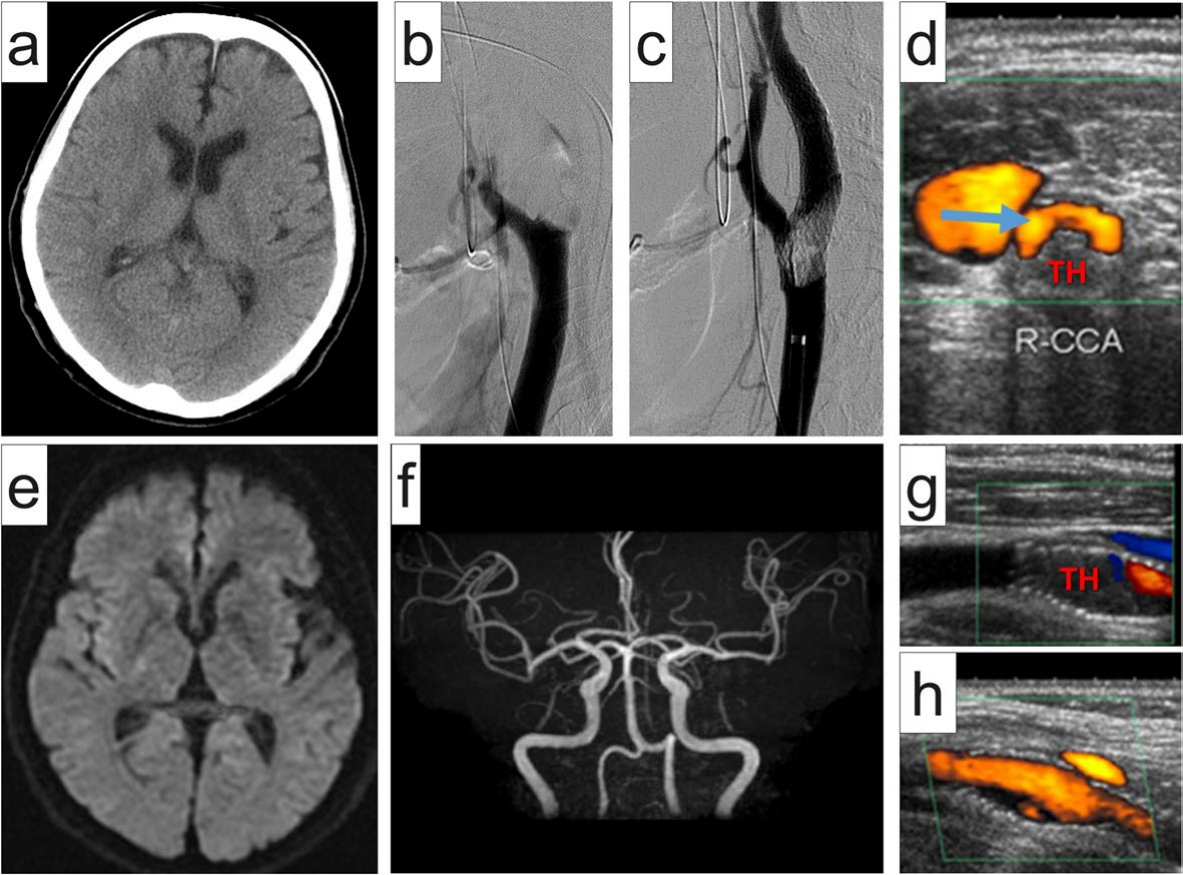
Imaging of the occluded right internal and external carotid arteries treated with endovascular stenting. a Head computed tomography image reveals neither intracranial hemorrhage nor early signs of brain infarction at the onset. b Angiography image shows that the bulb of the right internal carotid artery and the right external carotid artery is occluded by a giant thrombus. c The right internal and external carotid arteries are successfully revascularized by introducing a 10 mm × 40 mm PRECISE stent (Cordis, Miami, FL, USA) at the right carotid bifurcation. d Carotid Doppler ultrasonography image shows that the giant thrombus (TH) remains within the right common carotid artery, protruding from the stent. The blue arrow indicates the recanalized lumen. e Magnetic resonance image obtained on the day after recanalization shows no sign of cerebral infarction. f Magnetic resonance angiography image shows the patency of the vasculature (including the right internal carotid artery). g Carotid Doppler ultrasonography image shows that the thrombus (TH) remains within the right internal carotid artery 10 days after recanalization. h Carotid Doppler ultrasonography image shows that the thrombus has completely disappeared 67 days following recanalization
